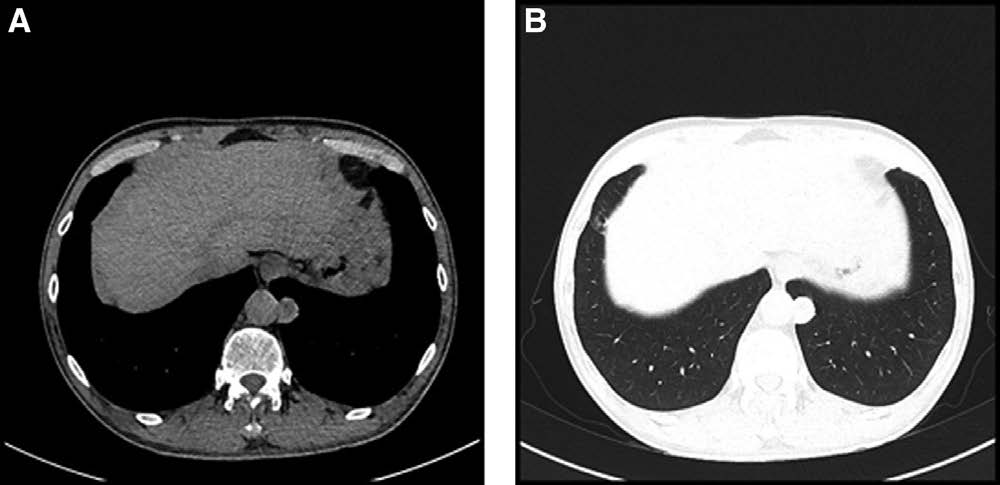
Computed tomography (CT) features of CCTL. (A, B) Contrast-enhanced CT tomography thorax-axial and coronal section showing the mass. (C, D) Contrast-enhanced CT tomography coronal section showing the mass. CCTLs = clear cell tumors of the lung, CT = computed tomography.
